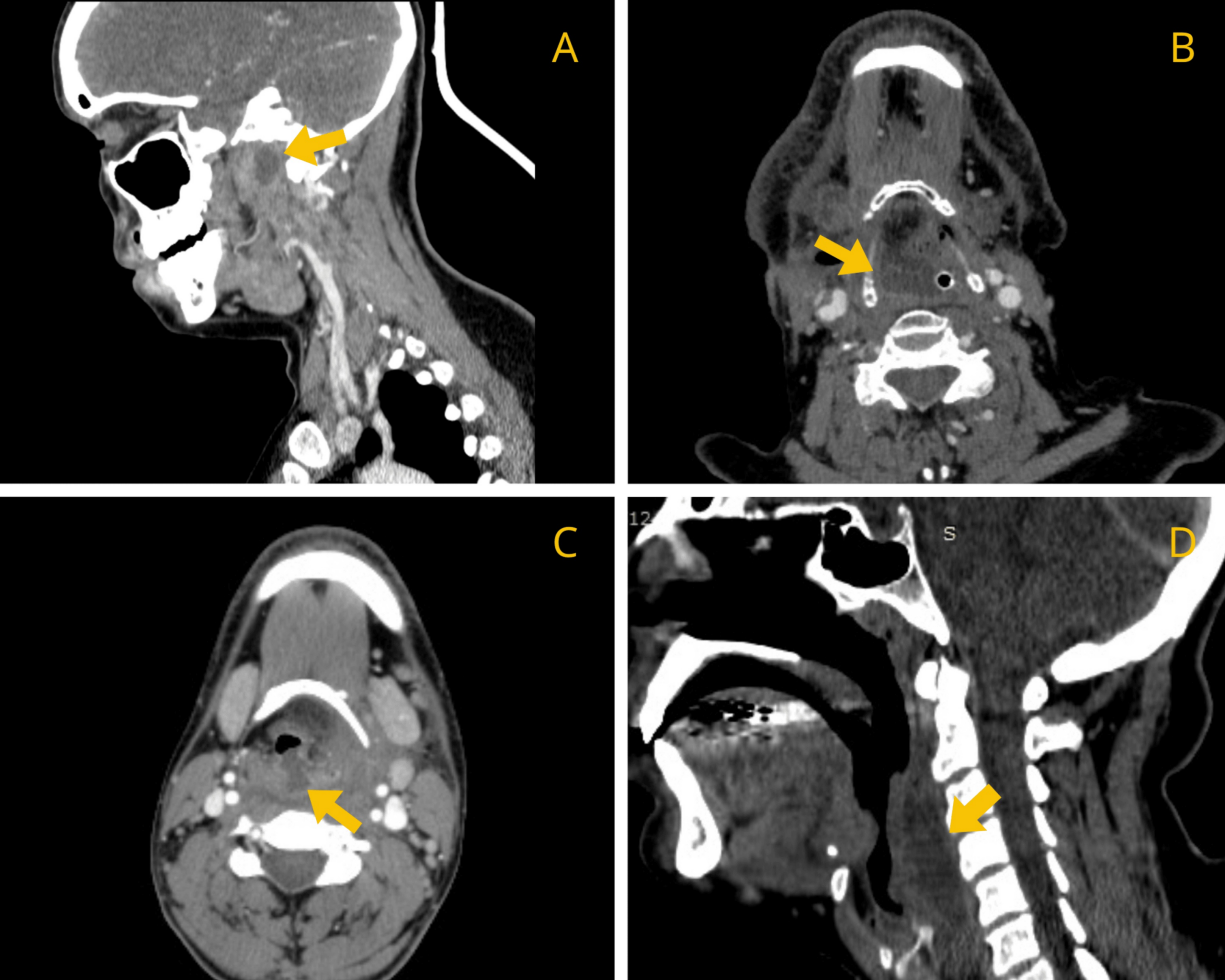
Contrast-enhanced CT images of patients 2 (A), 4 (B), 5 (C), and 11 (D) demonstrating RPA (yellow arrow). RPA: retropharyngeal abscess.
